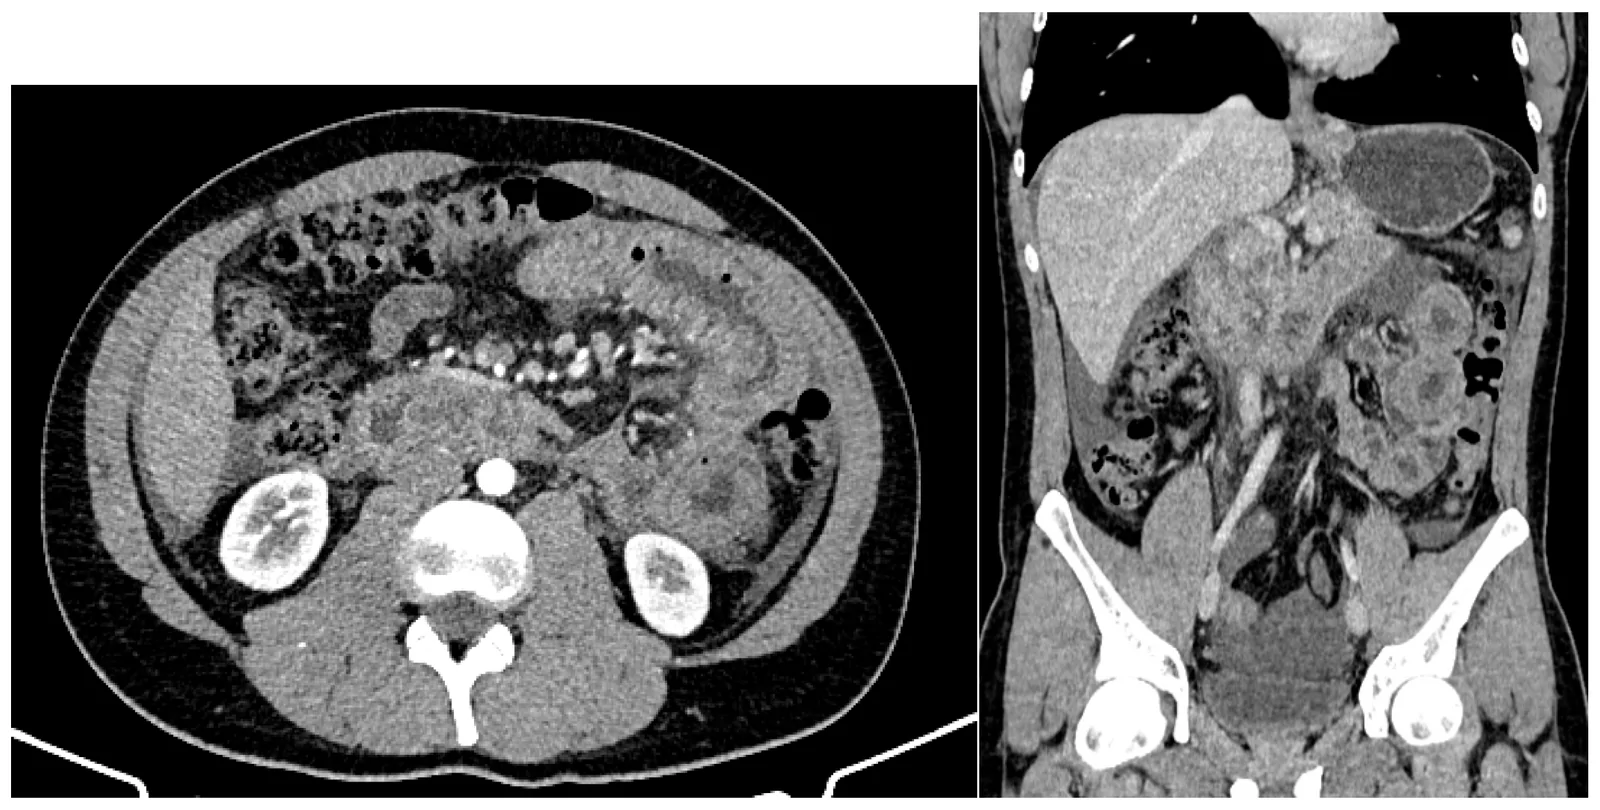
Contrast-enhanced abdomino-pelvic CT scan (axial and coronal reconstructions). Thickening of the duodenal and jejunal walls and intraperitoneal fluid.
